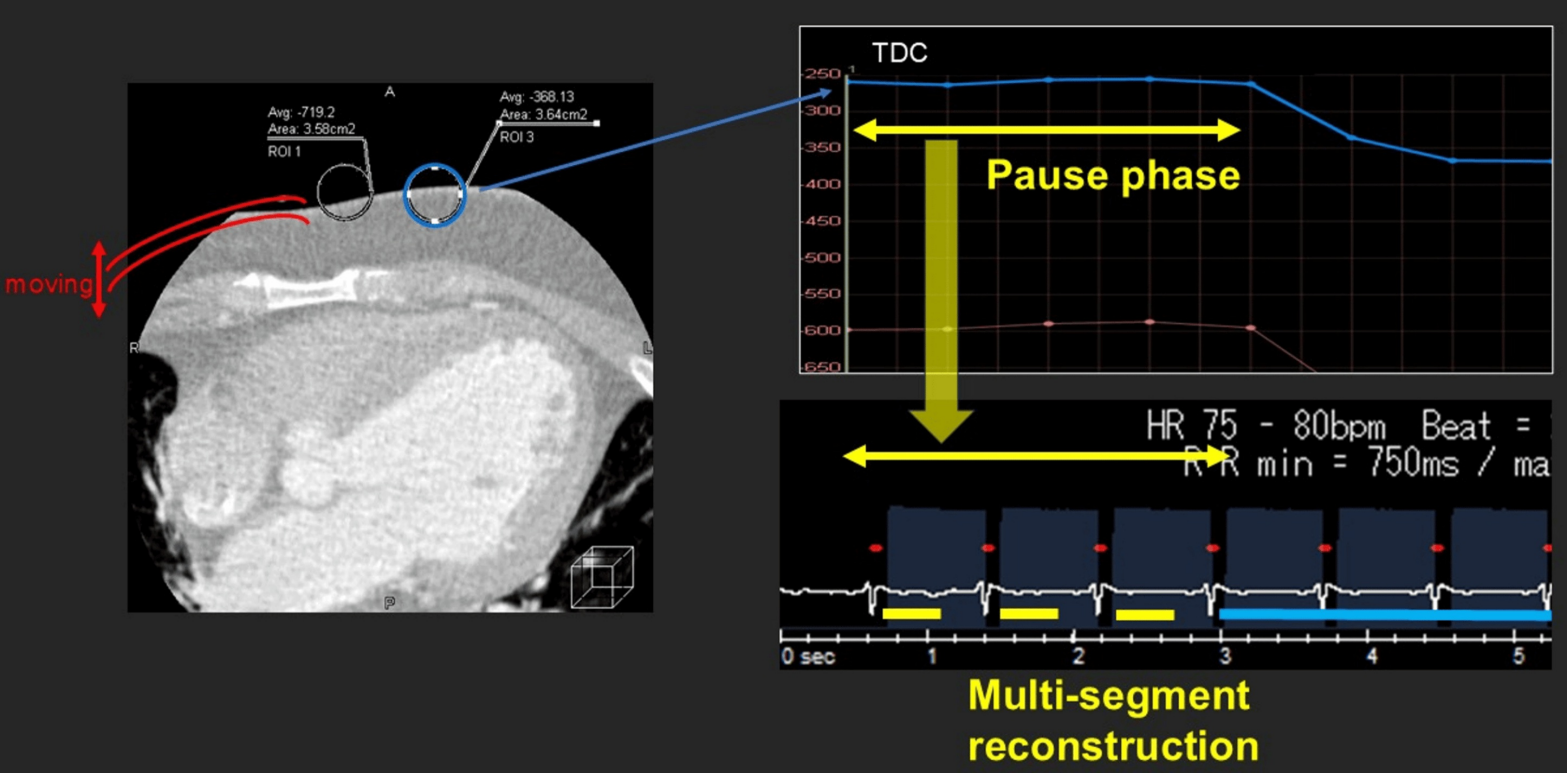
Identification of respiratory pause phases for FB-CCTA Respiratory pause phases were retrospectively identified using a TDC generated from a chest wall ROI. Time intervals corresponding to minimal respiratory motion were selected for subsequent respiratory phase–selective image reconstruction. FB-CCTA: Free-breathing coronary CT angiography, TDC: Time-density curve, ROI: Region of interest, HR: Heart rate, RR: Respiratory rate
